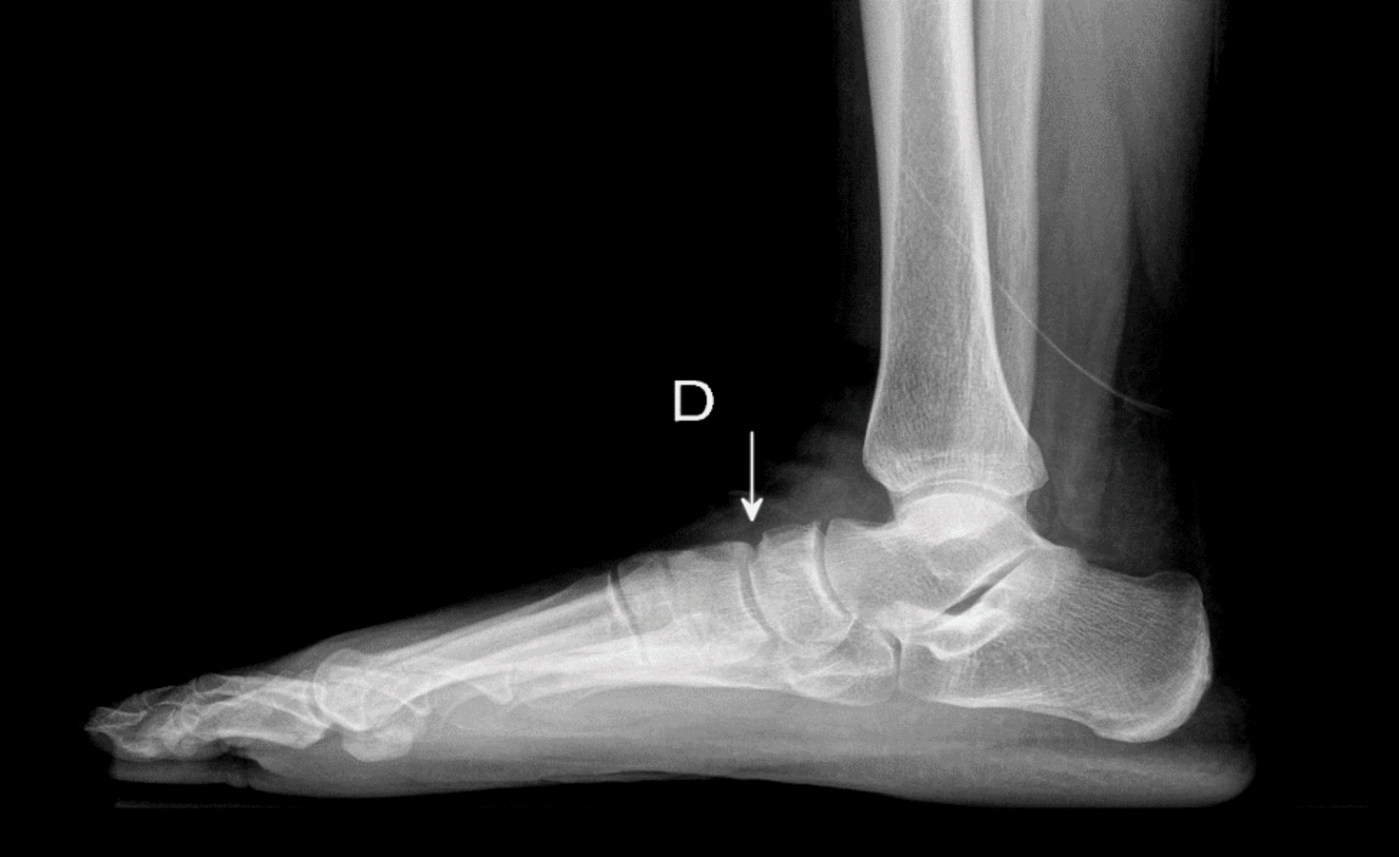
Weight-bearing lateral radiograph of the right foot Localized radiolucent area of the navicular bone (arrow), associated with early bone remodeling and mild flattening, consistent with stage 2 Müller-Weiss syndrome
